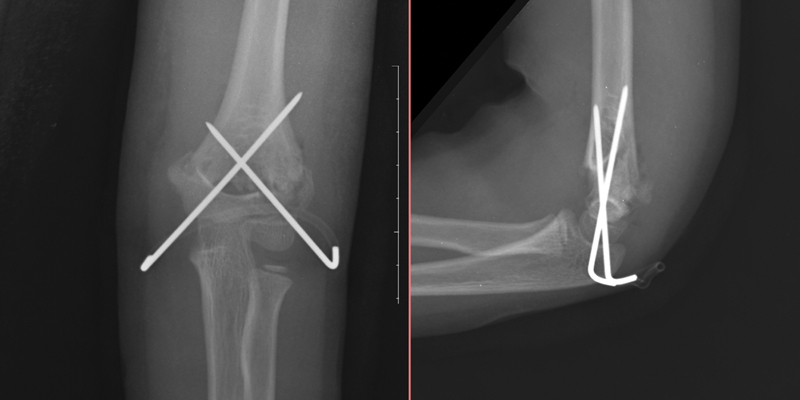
Anteroposterior and lateral radiographs of the distal humerus immediately after surgery.
